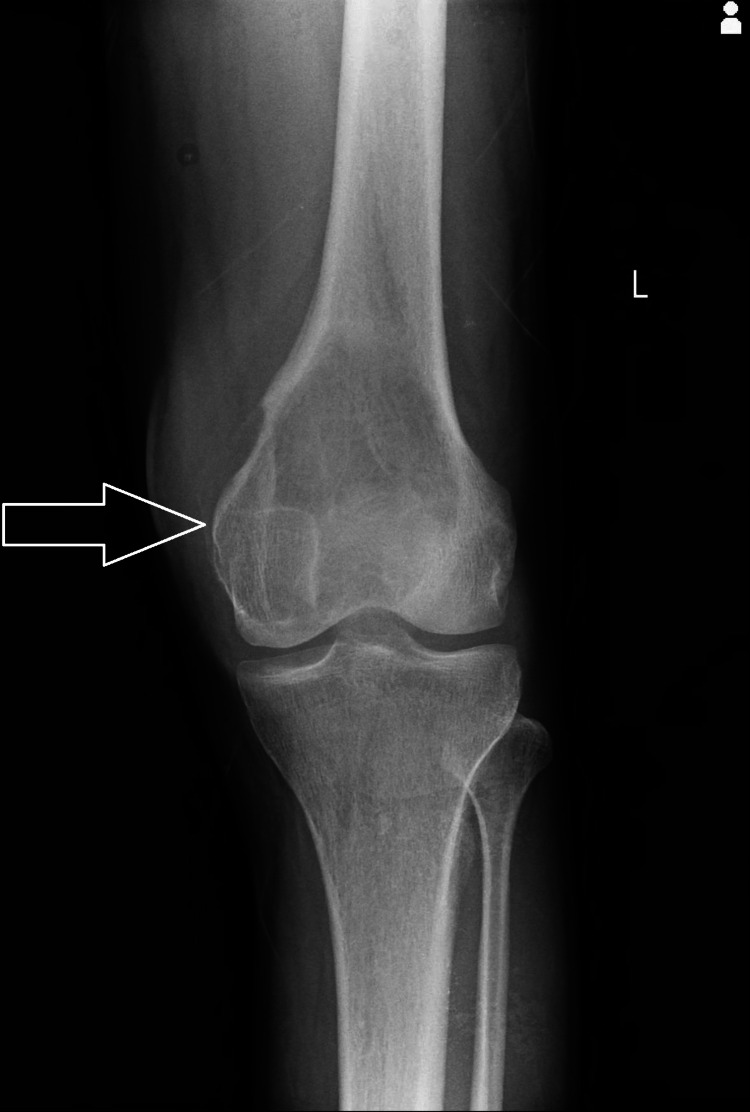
X-ray having homogenous radio-opaque area with a lytic appearance involving epiphysis of distal femur - anteroposterior view
